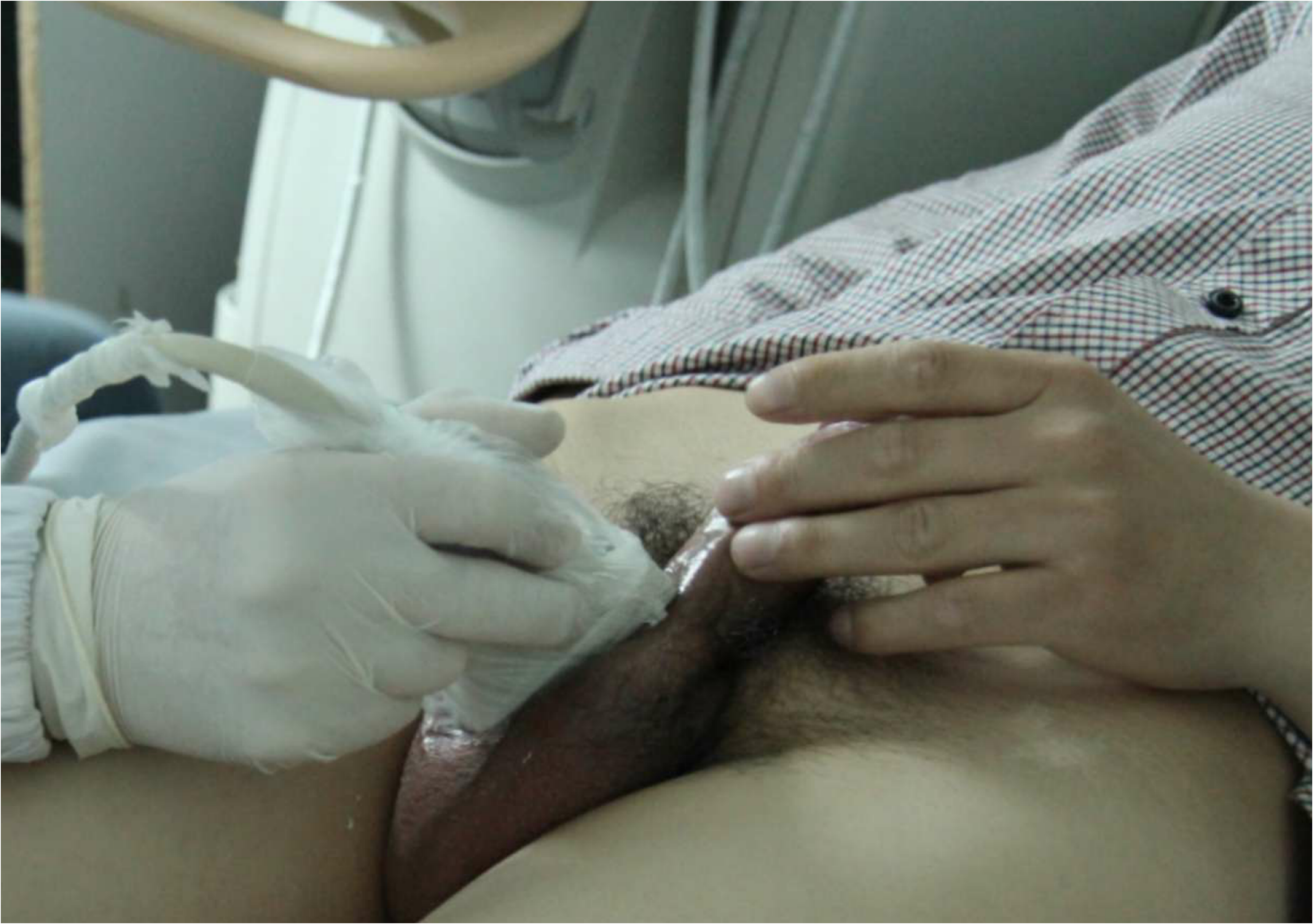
Actual operation photo of pharmaco‐penile duplex sonography.
